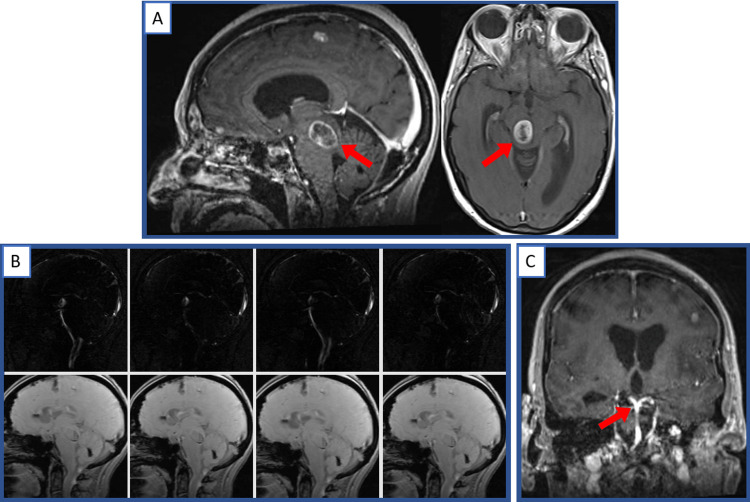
Preoperative imaging MRI with contrast demonstrating a 2cm ring-enhancing mass in the posterior right pons (arrows) compressing the cerebral aqueduct with moderate enlargement of the lateral ventricles visible on axial views (A). MRI flow study (B) showed no flow through the cerebral aqueduct. Additionally, the apex of the basilar artery (arrow) was in close proximity to the floor of the third ventricle (C). This figure was prepared for this submission using original images, and no credit/license is required.
